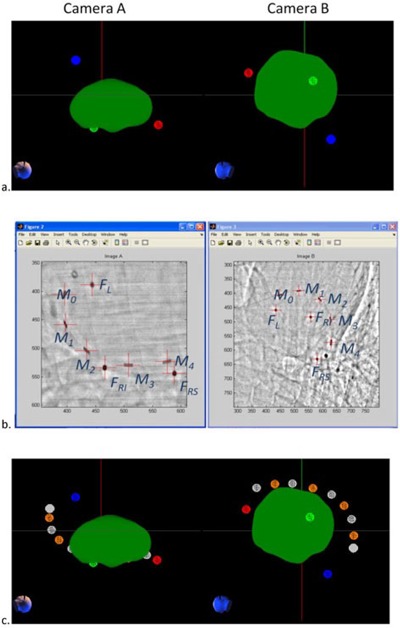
The method used to determine the dosimeter locations: (a) the locations of the sutured fiducials (red, blue, and green balls) in the CT image are projected to match the images from the CyberKnife system and show their spatial relationship to the prescription dose cloud (green); (b) enhanced CyberKnife X‐ray images showing the sutured fiducials ($F_{L}$, $F_{\text{RI}}$, $F_{\text{RS}}$) and the fiducials attached to the MOSFET flex ($M_{0}-M_{4}$); (c) positions of the MOSFET sensors (orange balls) relative to the fiducials on the MOSFET flex circuit (gray balls), the fiducials sutured to the epicardium (red, green, and blue balls), and the prescription dose cloud (green).
